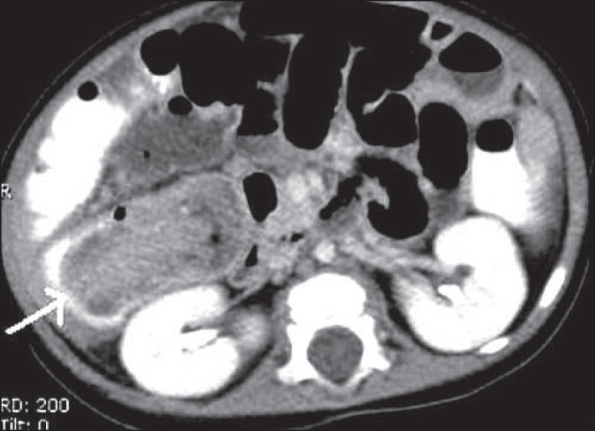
Ileocolic intussusception. Contrast-enhanced CT scan shows a ‘bowel in bowel’ configuration (arrow) with mesenteric fat invagination in the right lower quadrant
